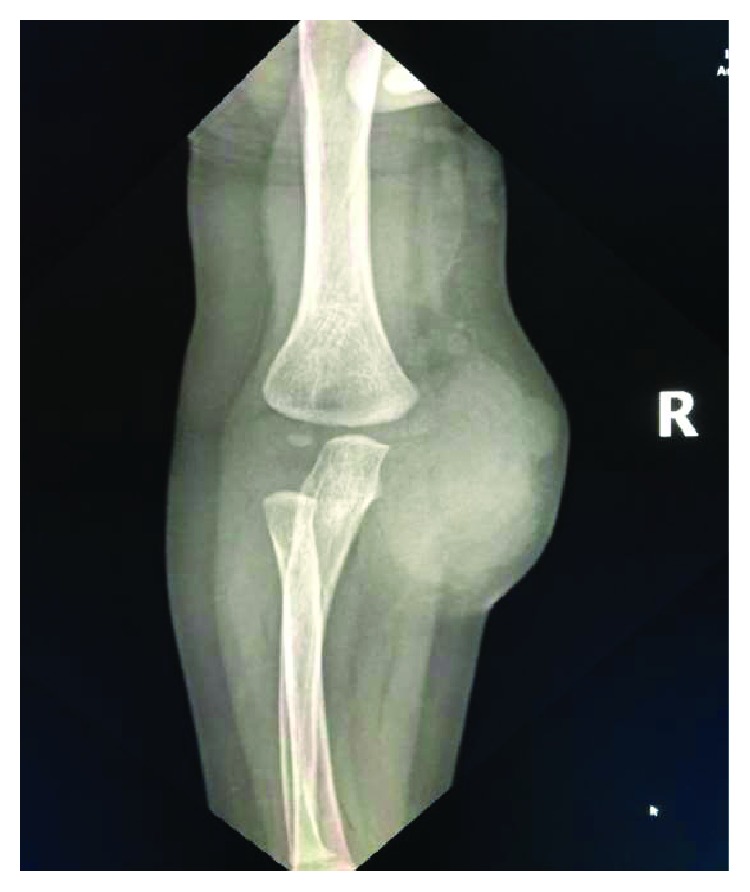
Plain radiograph AP view of the right elbow showed lobular soft tissue swelling suggesting a mass.
